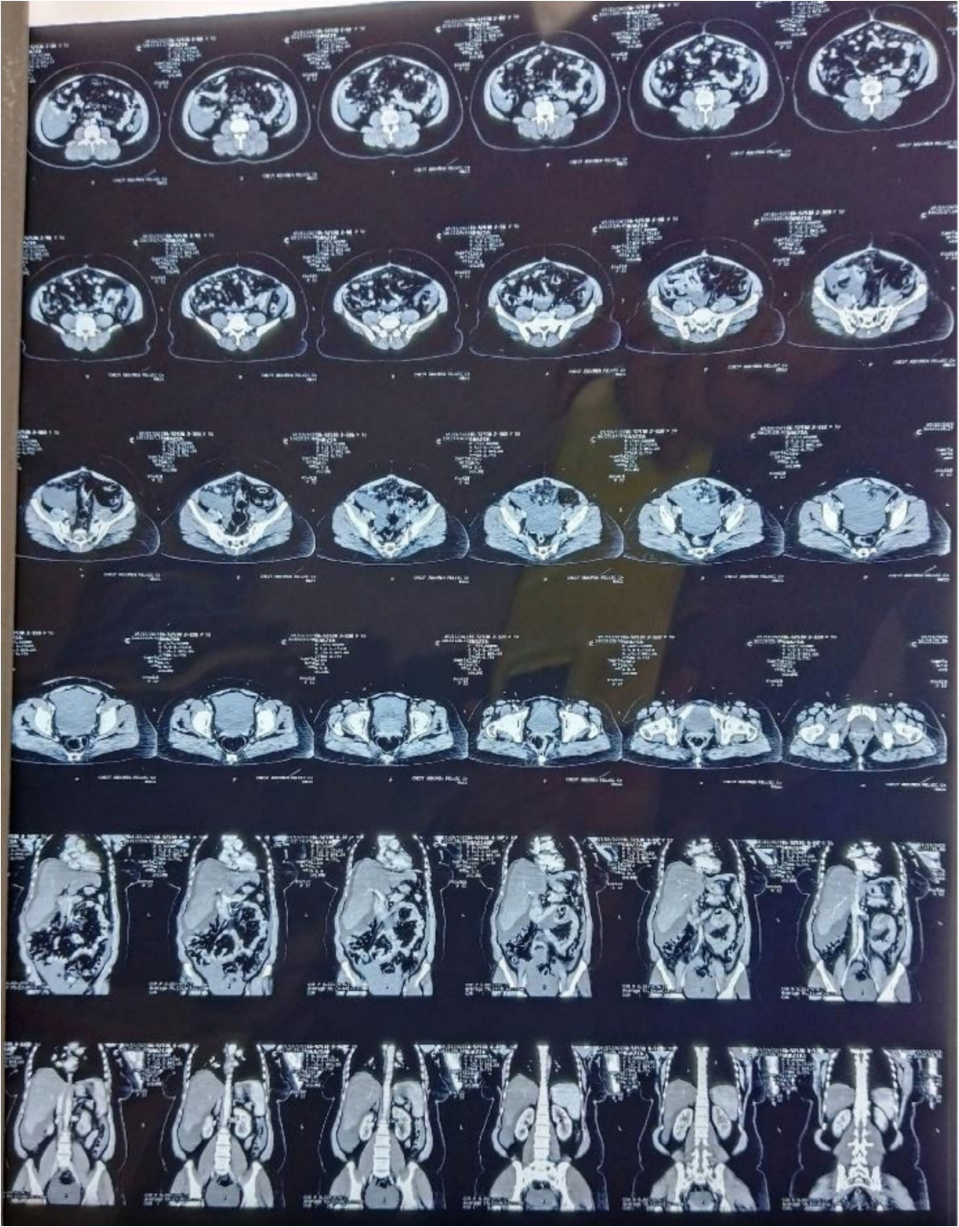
Follow‐up CT scan before carboplatin/etoposide.
